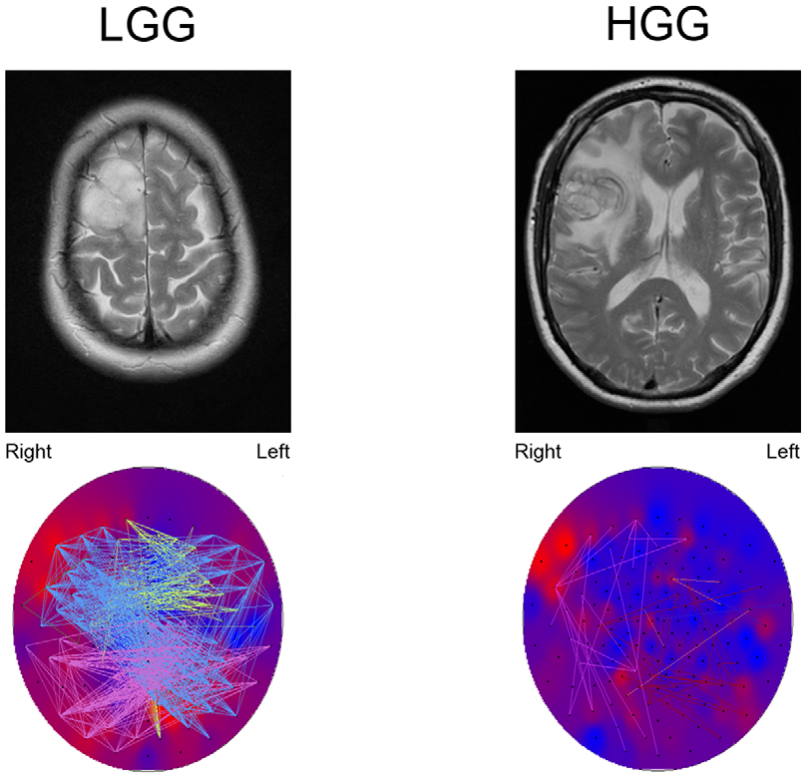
Example of theta band connection differences between a LGG patient and a HGG patient, both suffering from a tumor located in the right frontal lobe. The upper images show T2-weighted MRI images of the tumor. The lower images show theta band PLI levels (background colors; red colors represent high PLI levels, blue colors represent low PLI levels). Note that the tumor region seems to have the highest theta band PLI. The colored lines represent connections between sensors, each color representing another module. Connections are shown when their strength passes an arbitrary threshold chosen for optimal connection visualization. In HGG patients, only few connections exist above the threshold. Note that especially connections to the tumor region in LGG patients pass the threshold. However, two other modules are also clearly shown that are not found in the HGG patient, suggesting that the differences between LGG and HGG patients networks are not restricted to the tumor region.
